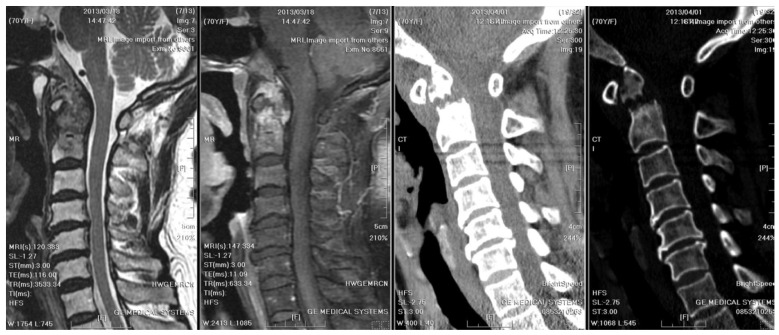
Initial MRI and CT of the cervical spine: They revealed an osteolytic lesion at C2, along with prevertebral and paravertebral soft tissue infiltration. Mild spinal canal stenosis was observed, while the diameter of the spinal cord was within an acceptable range. Bony destruction of C2 odontoid process and atlantoaxial subluxation were identified. Heterogeneous contrast enhancement of vertebral body and subligamentous spread at prevertebral area were observed. The wall of abscess was ill-defined, smooth, and regular. However, the thickness of the wall was equivocal.
